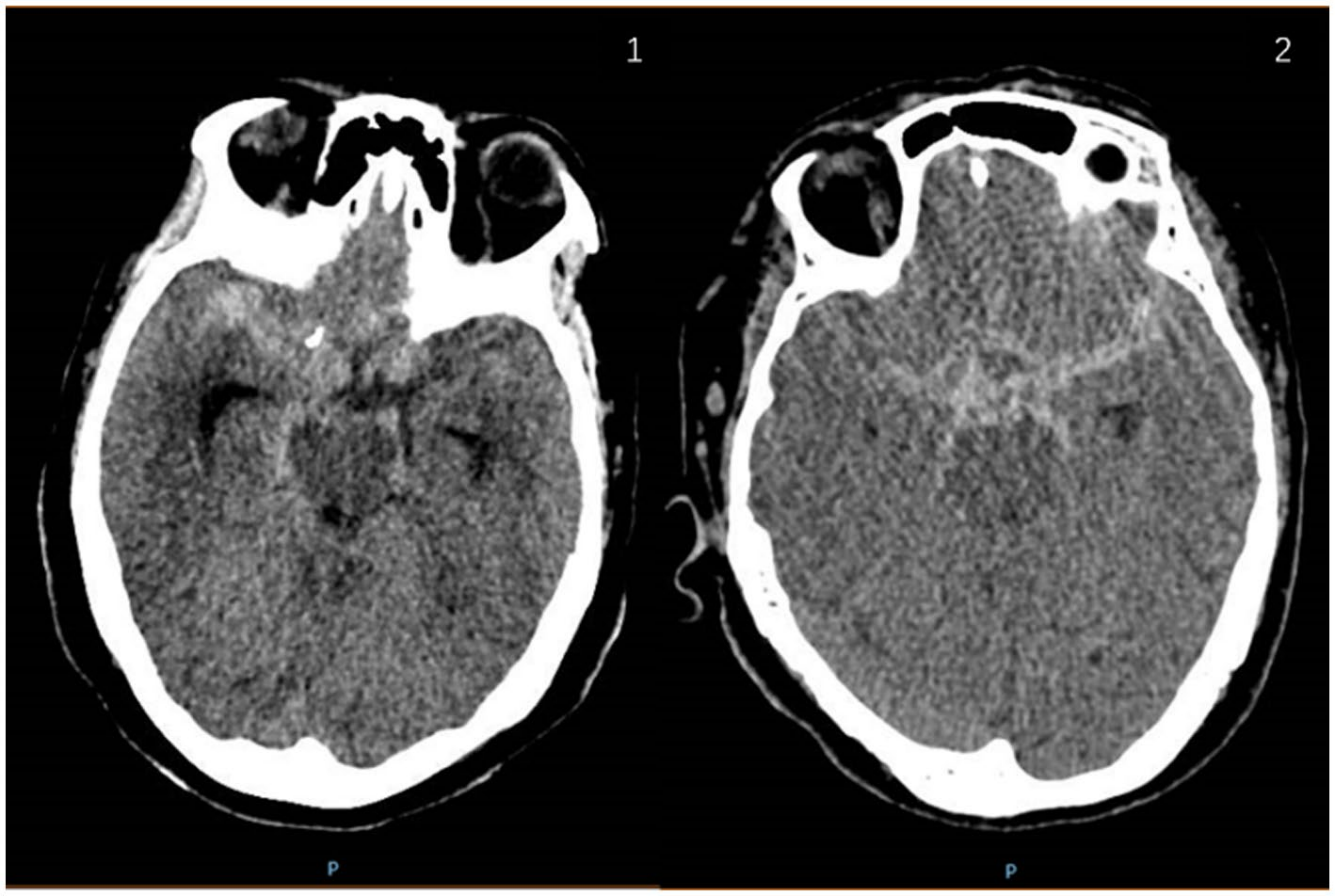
Head CT images of case 1 and case 2 with ruptured aneurysm and subarachnoid hemorrhage. 2.1: case 1; 2.2: case 2.
